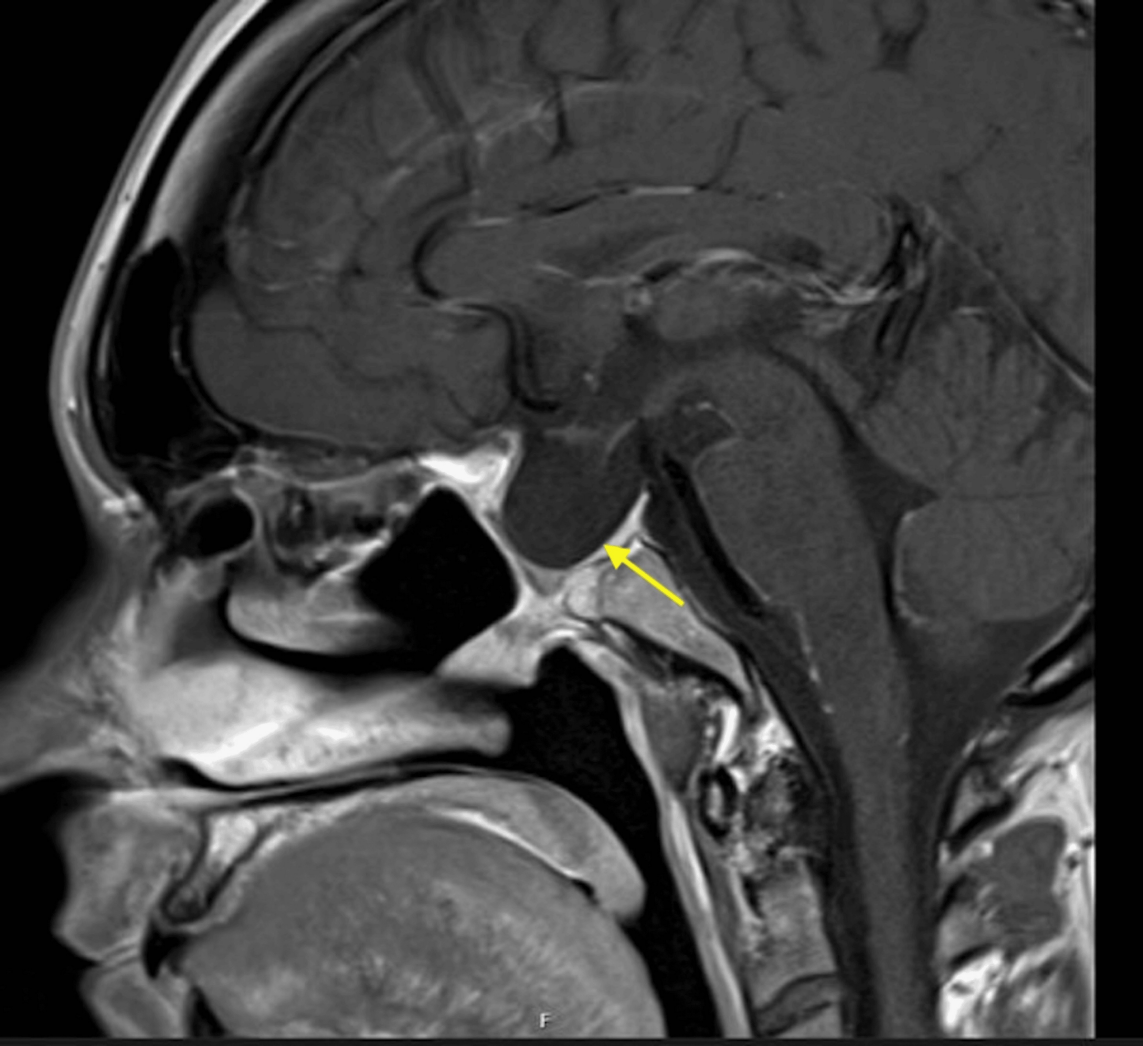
Sagittal view of the postoperative pituitary MRI Arrow pointing towards a partial empty sella after complete excision of a pituitary macroadenoma.
